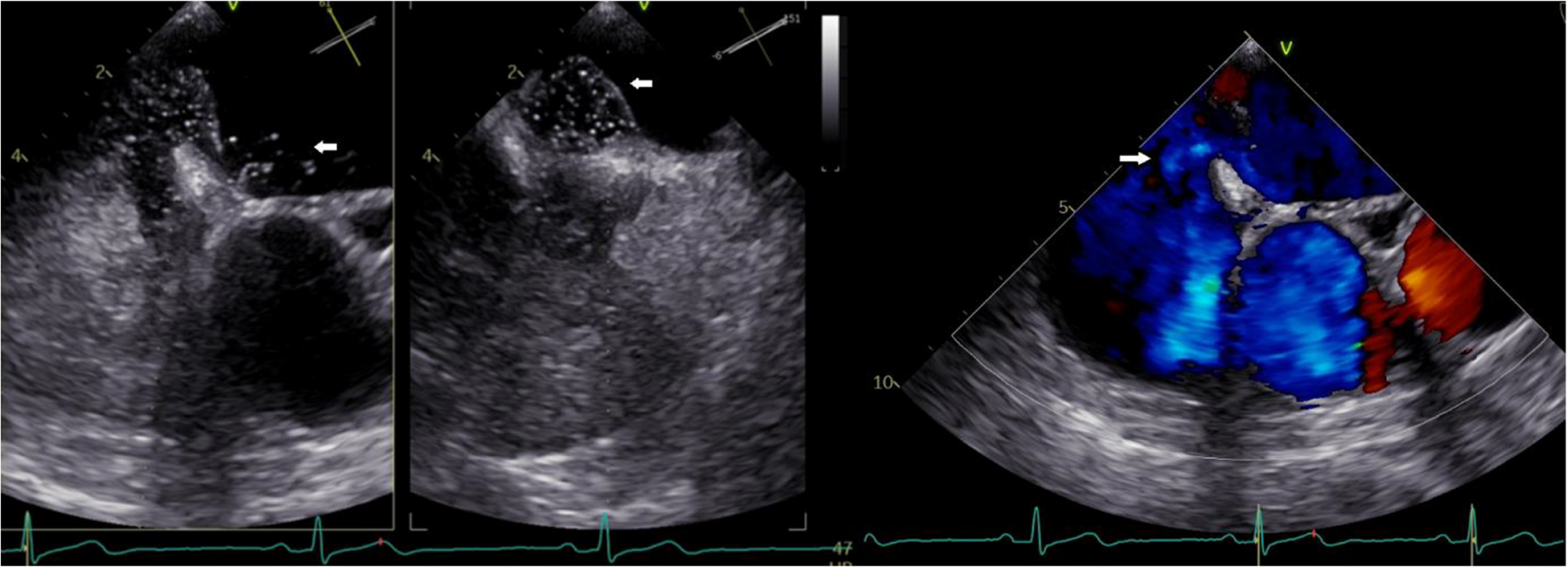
Echocardiography showing a PFO using agitated sodium chloride contrast (left) and on colour doppler (right)
